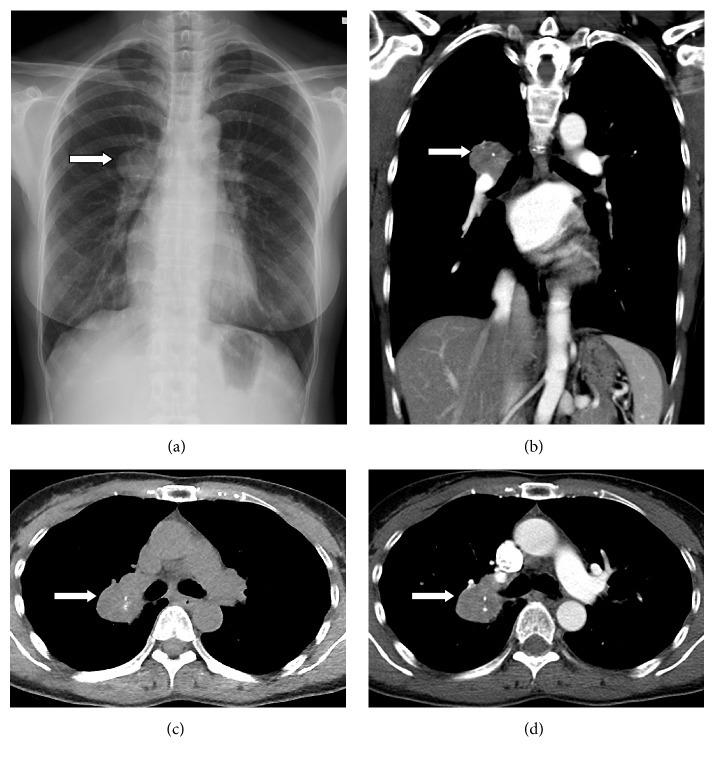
(a) Chest X-ray study detects a mass (white arrow) in the right pulmonary hilar region. (c) Precontrast CT scan reveals a well-defined right hilar mass with flecks of calcification and some low attenuation area. ((b) and (d)) Contrast enhanced CT scan shows a heterogeneous enhanced mass in the right pulmonary hilar region.
